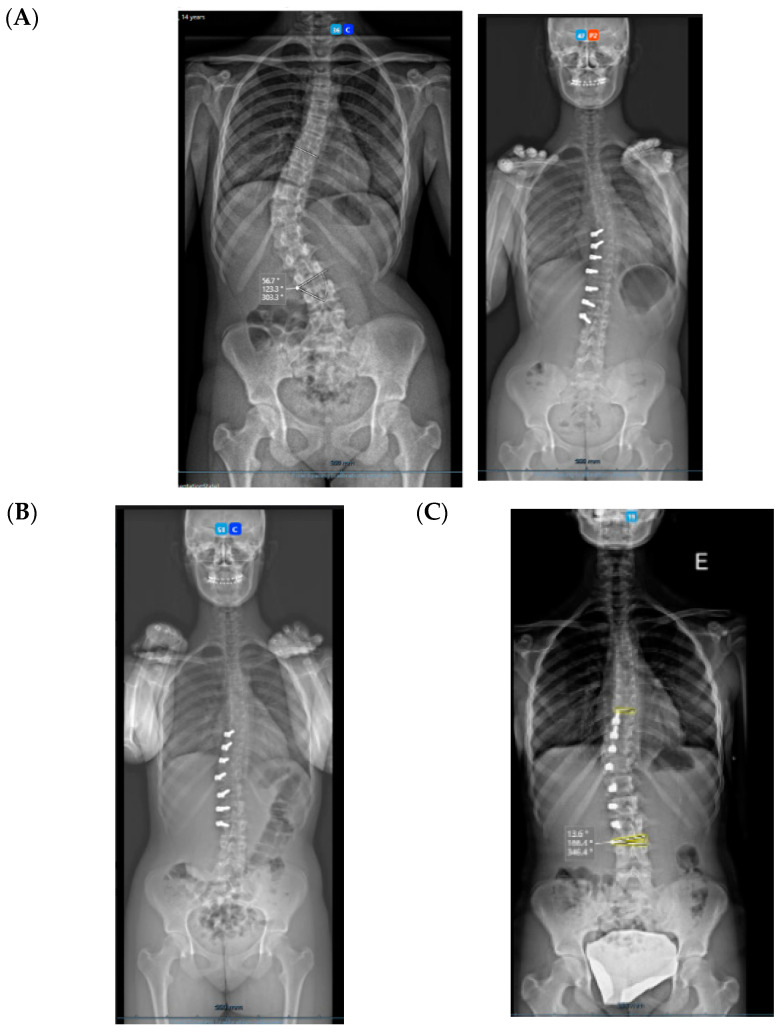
Over-correction. (A) Pre-op (left) + 18-month FU (curvature apex corrects to the opposite side) (right). (B) Post tether release. (C) Immediate post-op erect radiograph.
